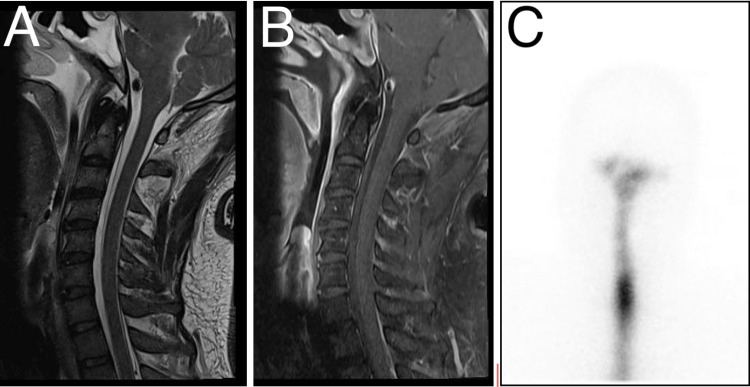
Cervical MRI CISS protocol in (A) T2 and (B) contrast sagittal views showing a longitudinal CSF collection in the anterior extradural space from the level of C2 down to the level of T1, effacing the thecal sac. (C) Radionuclide cisternography showing no diffusion of radioisotope in the extra-arachnoidal space. CISS, constructive interference in steady state; CSF, cerebrospinal fluid
